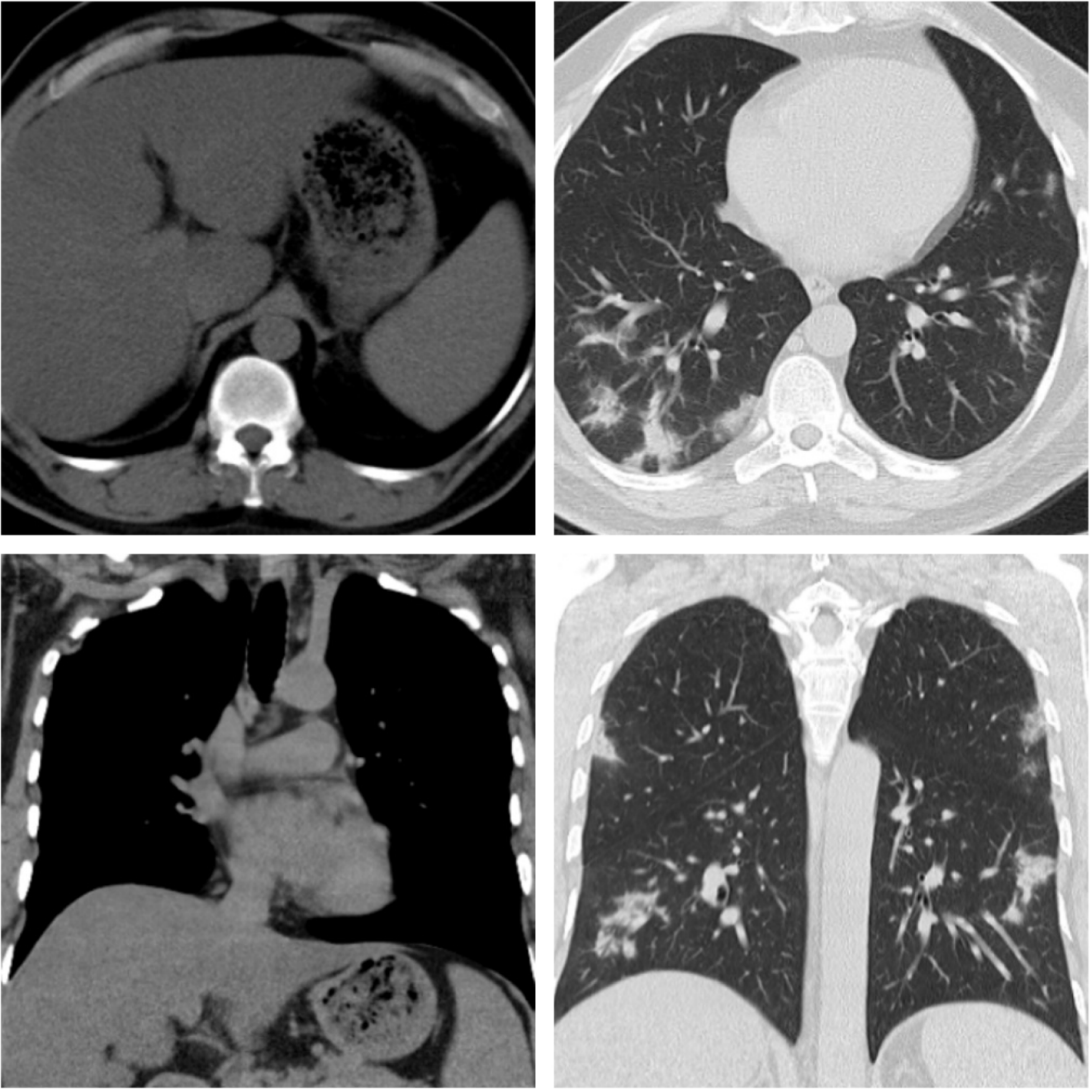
Male patient, 45 years old. CT chest shows multiple bilateral pulmonary patchy ground-glass opacities, reported as CORADs 5. Upper abdominal cuts show hepatic steatosis
